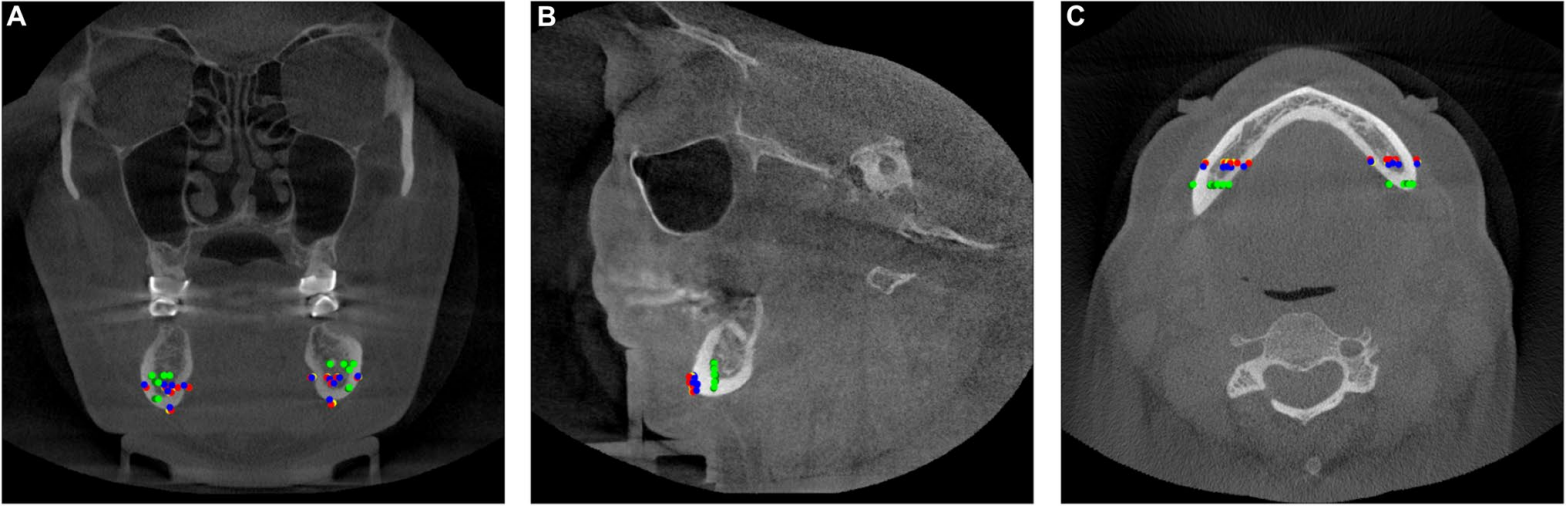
Sample of CBCT image with localized landmarks in the osteotomy line of the BSSO in coronal (A), sagittal (B), and axial (C) MPR views; red landmarks: GT; lime, green, yellow landmarks: experts 1, 2, 3; blue landmarks: AI
